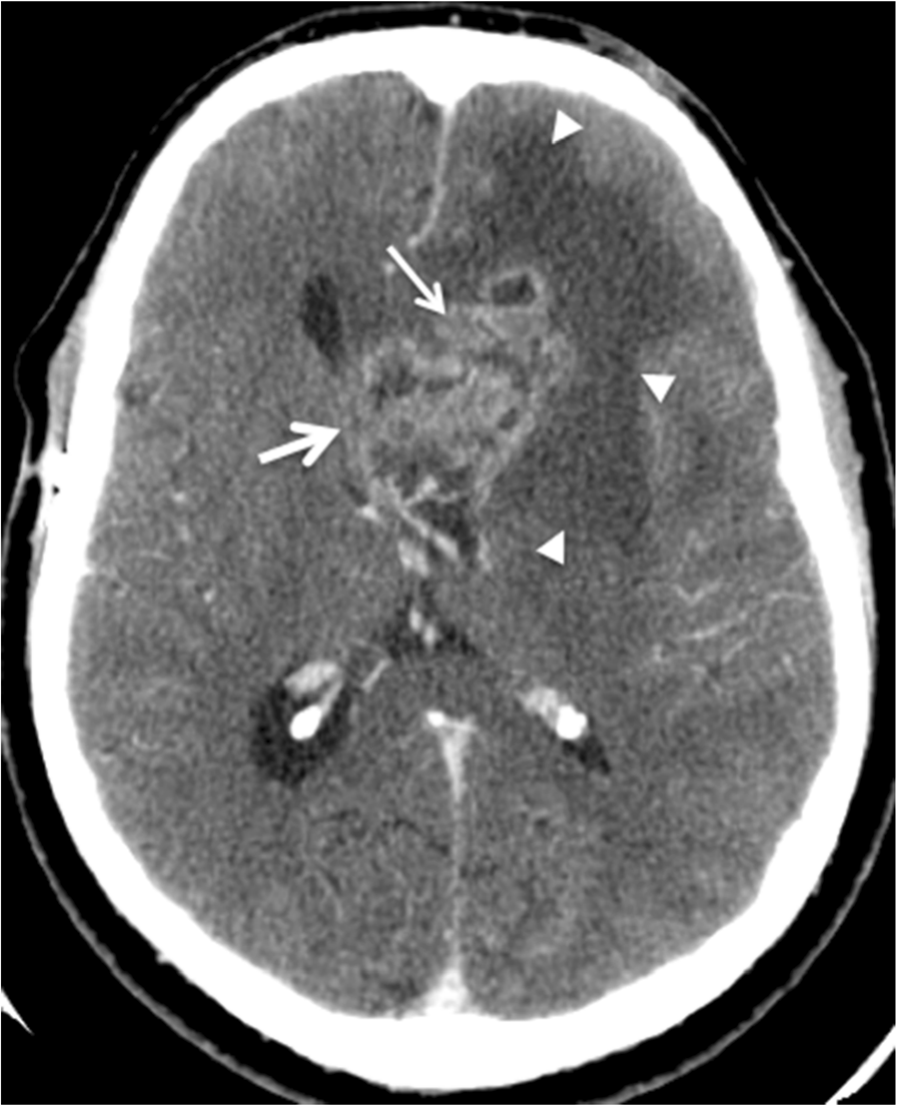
A 37-year-old man with intracranial tumor with mass effect. Axial post-intravenous contrast images. Computed tomographic images of a patient with glioblastoma multiforme in the left frontal lobe. The lesion with irregular contours and heterogeneous enhancement (thin arrow) has mass effect characterized by hypoattenuation (edema) of the adjacent white matter (arrowheads), compression of the lateral ventricle, and contralateral deviation of the medial line structures, with signs of subfalcine herniation (thick arrow).
